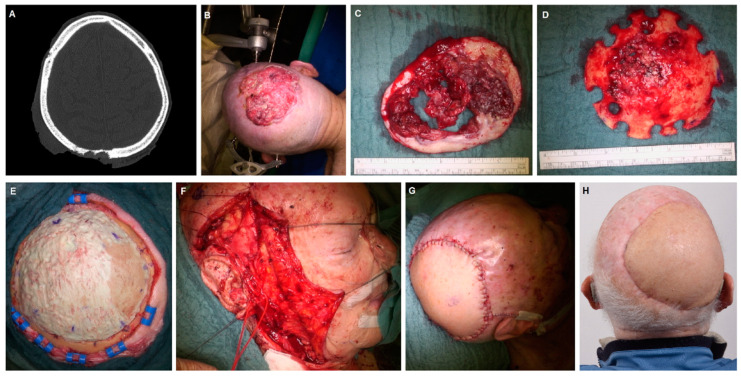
Example of a cranial reconstruction using an ALT flap in a patient with a cutaneous squamous cell carcinoma of the scalp. (A): CT scan presenting the primary tumor region with infiltration of the calvarial bone. (B): Image of the intraoperative situation before tumor resection. (C): Resected soft tissue. (D): Resected calvarial bone. (E): Intraoperative situation after coverage of the osseous defect with Polymethyl methacrylate (PMMA). (F): Image of the intraoperative situation after anastomosis of the pedicle vessels to the facial artery and the facial vein. (G): Intraoperative situation after soft tissue reconstruction with the ALT flap. (H): Postoperative situation two months after surgery.
